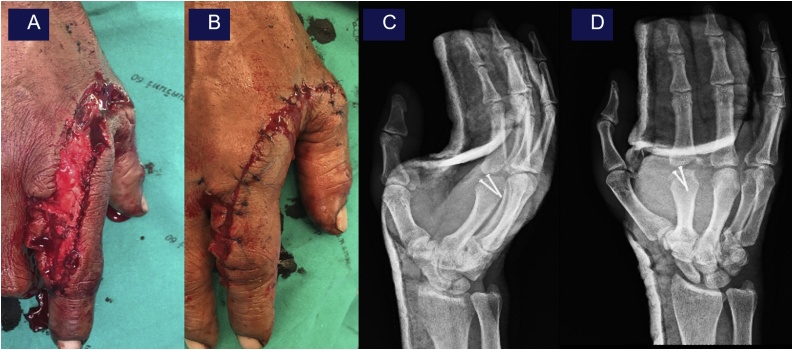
Intra-operative repair collateral ligament complex (Fig. 3A-B) and post – operative showing post-operative x-ray oblique view (Fig. 3C) and AP view (Fig. 3D).
